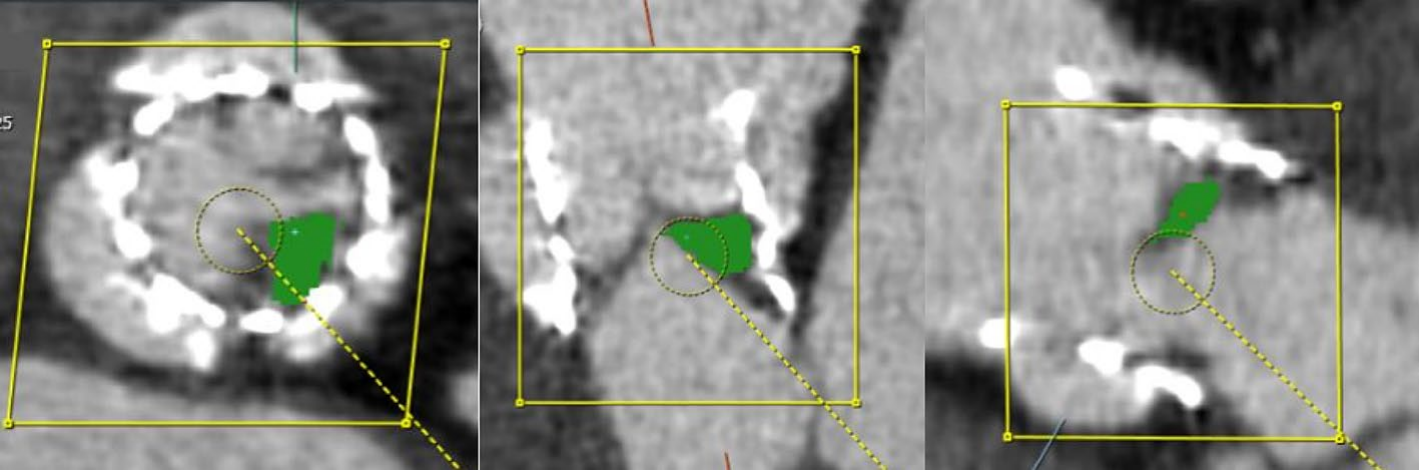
Cardiac CT images of an 83-year-old female exhibiting leaflet thrombosis of the bioprosthetic aortic valve. An ROI is drawn along the hypoattenuated leaflet thickening of the bioprosthetic aortic valve (green color). CT, computed tomography.
